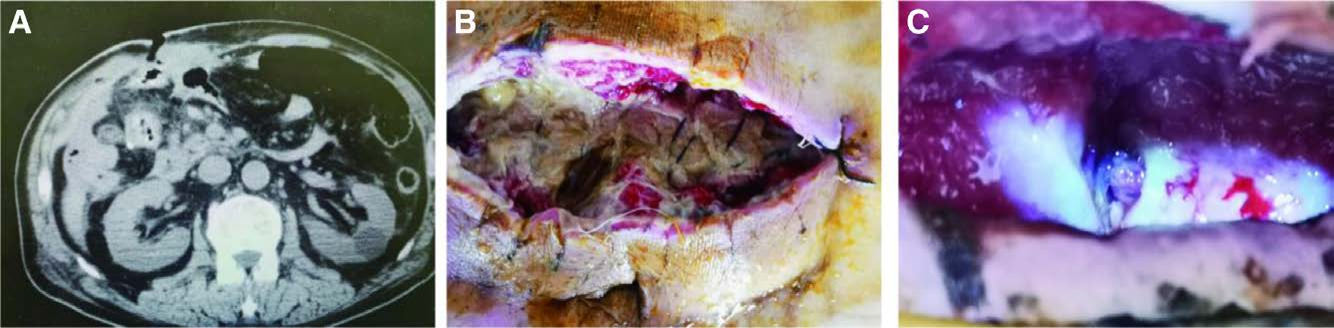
CT images and incision changes. (A) enterocutaneous fistula occurs with a distinct sinus tract, no abscesses seen. (B) Incision split with necrotic tissue attached to the base. (C) enterocutaneous fistula at the base of the incision, with leakage of digestive fluids. CT = computed tomography.
